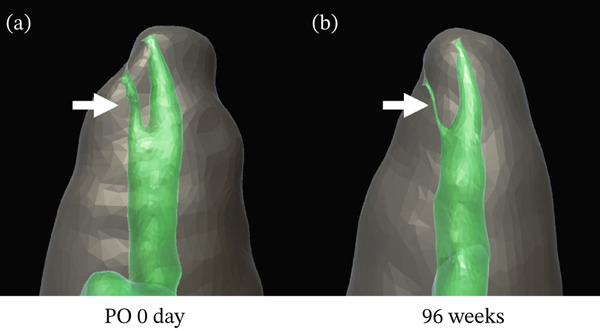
Case 2. Three‐dimensional reconstructed CBCT images of the apical region. (a) Immediately after cell transplantation (postoperative day 0). (b) At 96 weeks postoperatively. Arrows indicate the perforation site on the labial aspect of the apex. Clinically relevant finding: progressive narrowing and mineralization localized to the perforation site, whereas the original apical foramen remains relatively unchanged. This pattern suggests localized mineralized tissue formation at the perforation site rather than diffuse apical closure.
